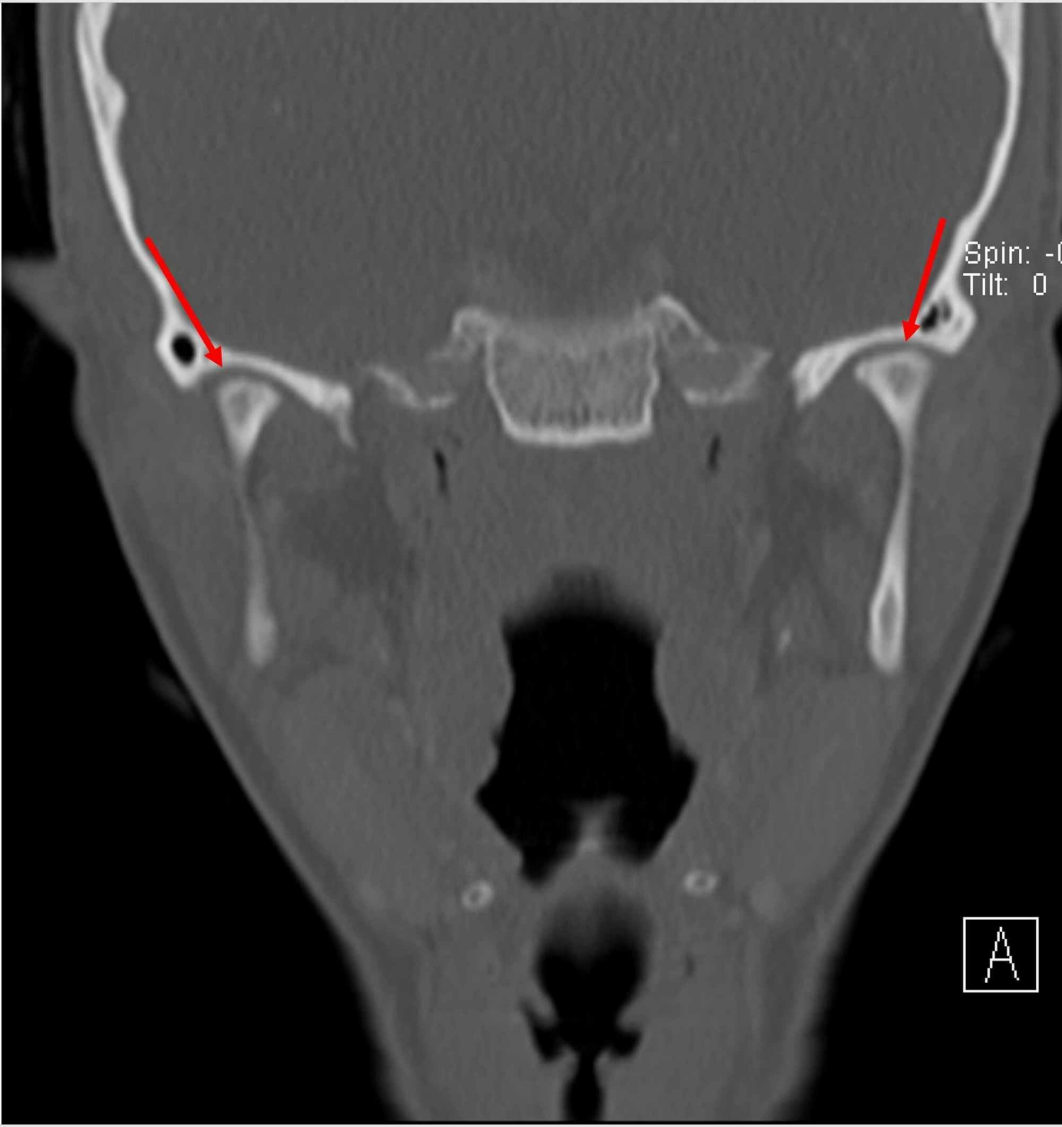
Coronal maxillofacial computed tomography (CT) (bone window) demonstrates resorption/flattening of the bilateral mandibular condyles and joint space narrowing of the bilateral temporomandibular joints
